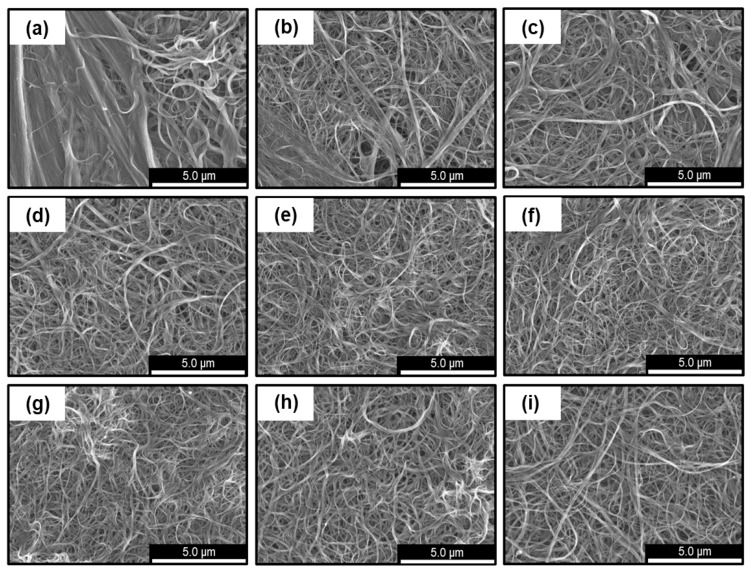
Surface morphologies of SWCNT films with different ultrasonic dispersion amplitudes in the SWCNT ink production. (a) 10%, (b) 20%, (c) 30%, (d) 40%, (e) 50%, (f) 60%, (g) 70%, (h) 80%, and (i) 90%.
